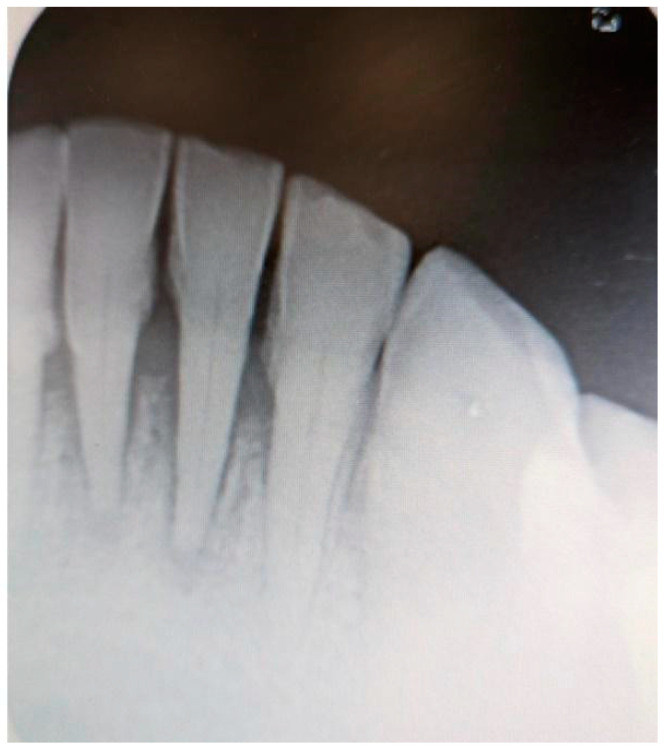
Digital periapical radiograph of the patient’s mandibular incisors.
